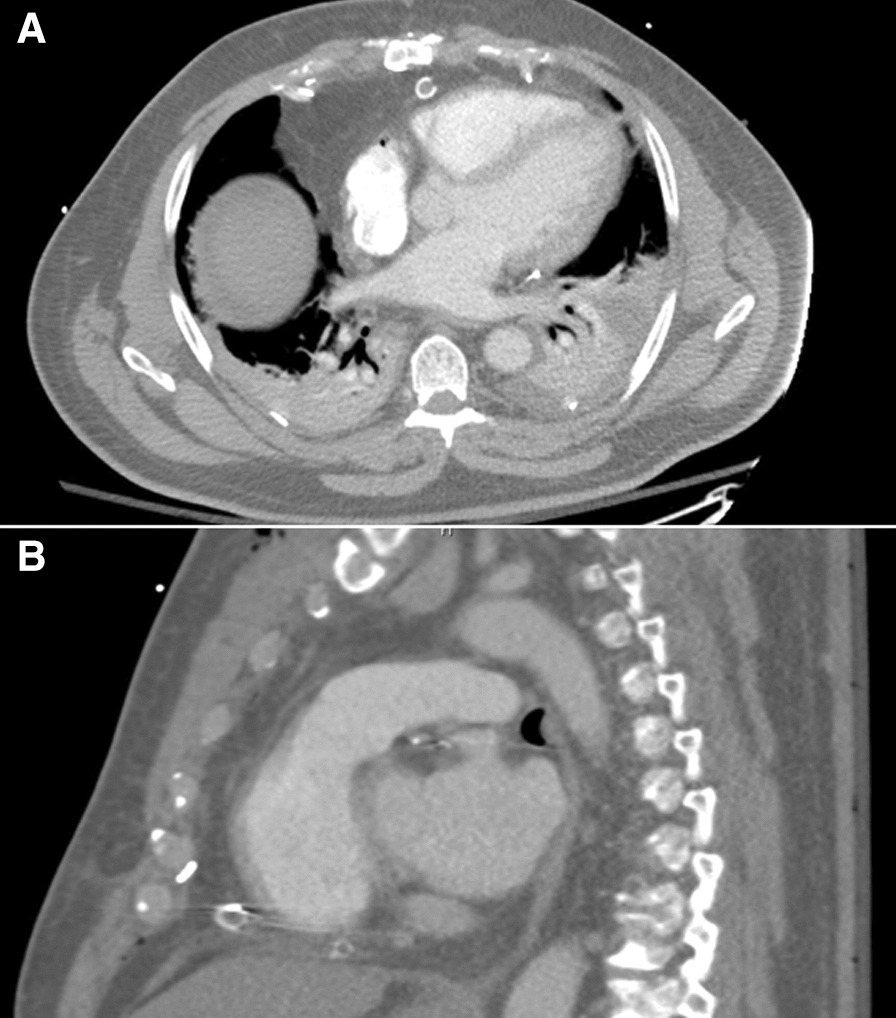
A Axial and B sagittal computed tomography images demonstrating large amounts of soft tissue around the right ventricle and right atrium
